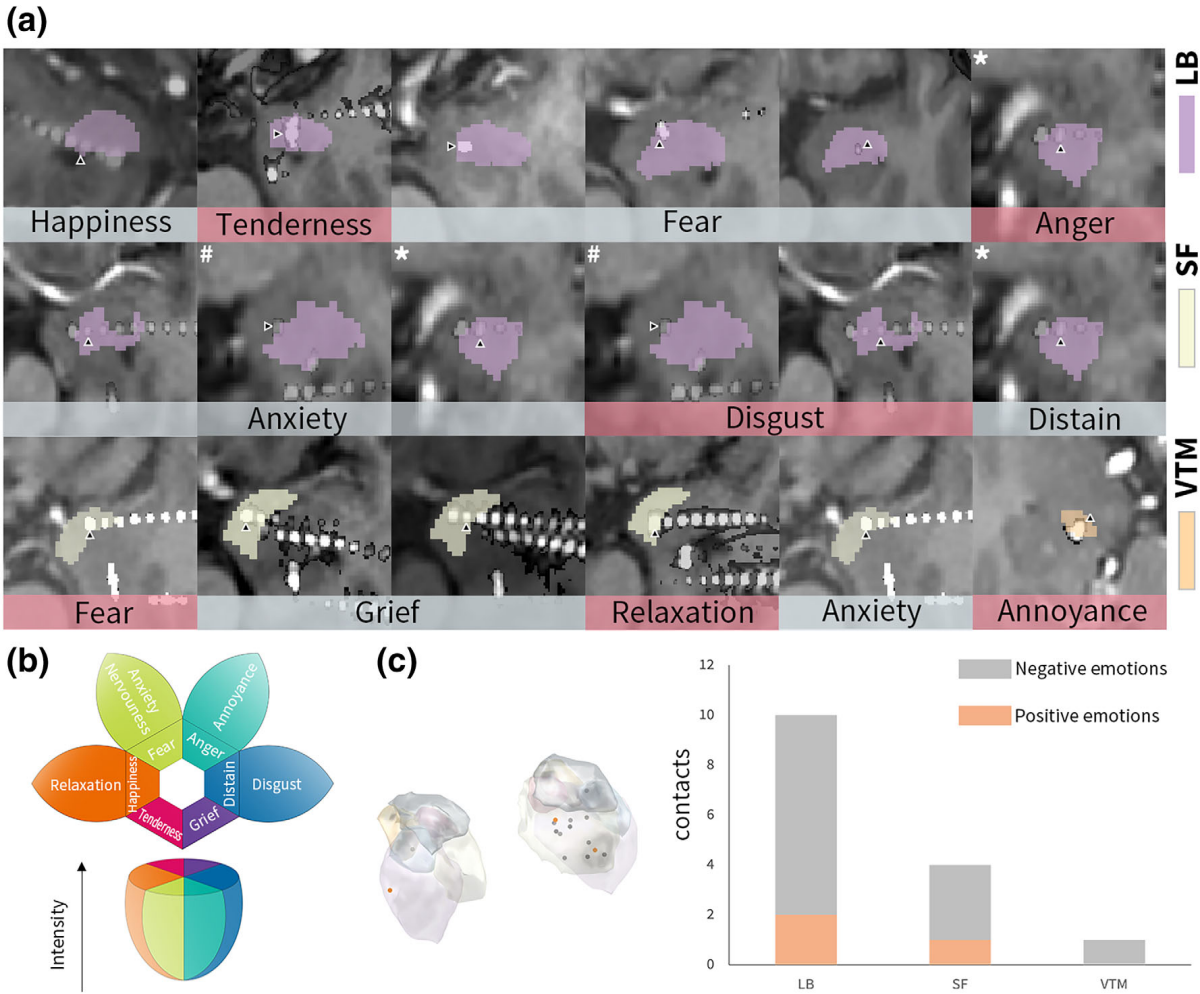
Precise localization of stimulation sites and the evoked emotional responses. (a) The reconstruction of electrodes with postoperative CT images registered in preoperative MRI images. The black triangles indicate the stimulation site. The masks with different colors show the amygdala subnuclei. (b) The emotion wheel of the evoked emotional experiences. (c) The three‐dimensional distribution of stimulation sites of the amygdala with either positive or negative emotions (left) and the emotion distribution in the level of subnuclei (right).
